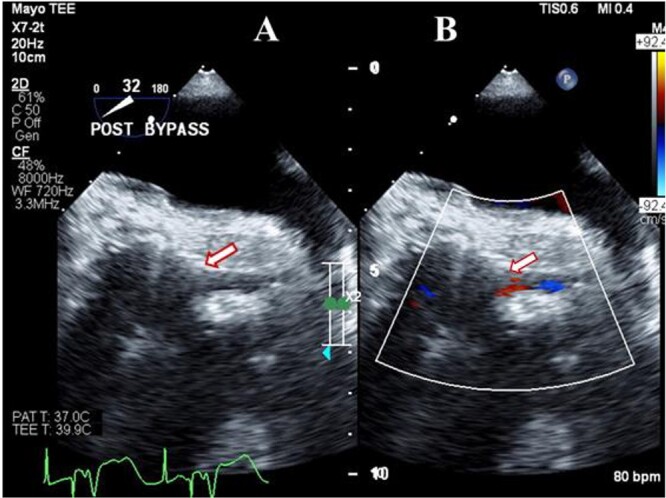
Transesophageal image following Bentall operation showing the widely patent (arrow) left main coronary artery (A) with Laminar flow (arrow) on color Doppler imaging (B).
